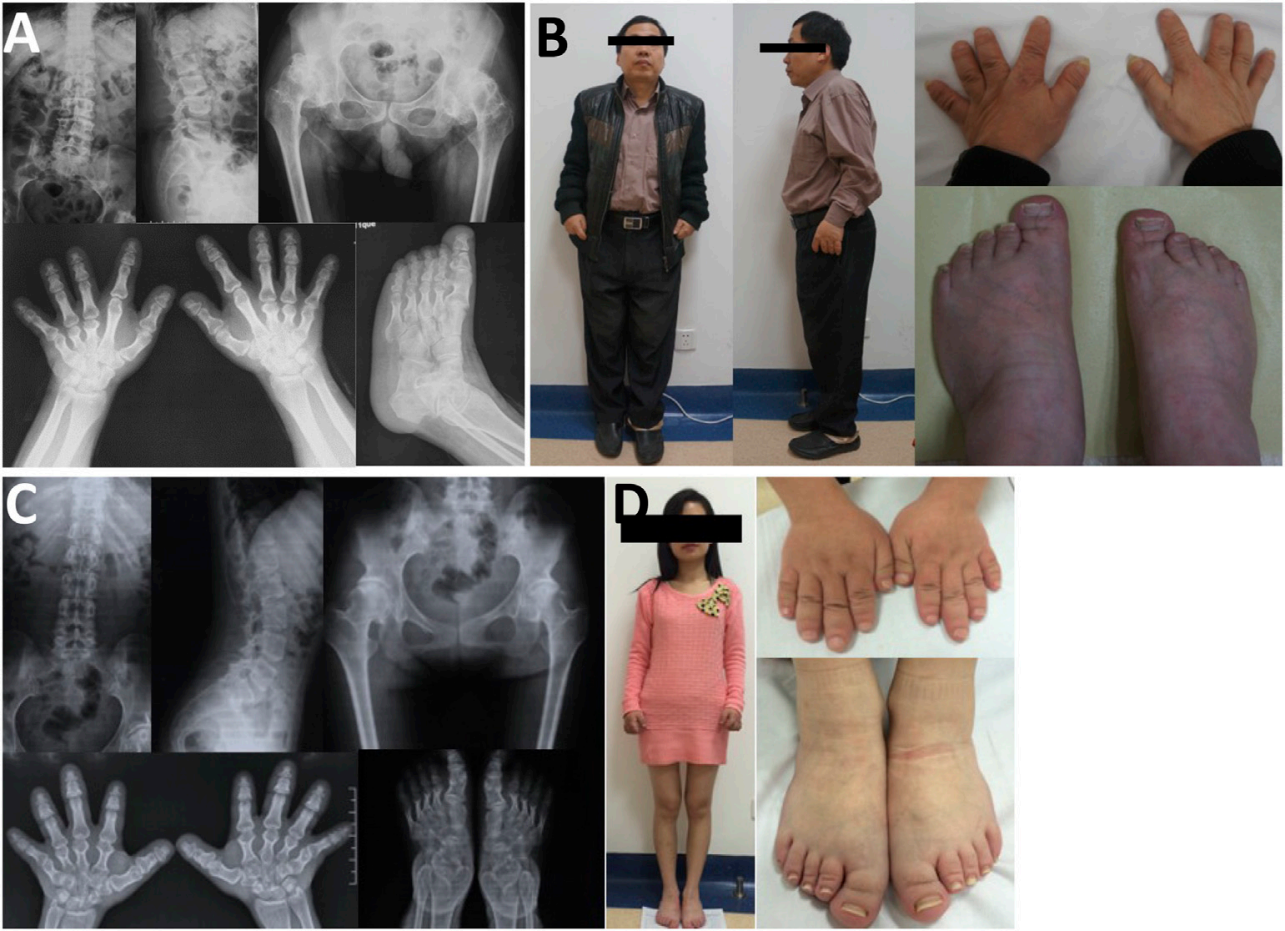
Photographs and radiographs of the family 7. (A,B) Radiographs of the spine, pelvis, hands, and feet (A) and photographs (B) of proband 7 (Ⅱ-2) in family 7. (A) Mild flattening and irregular shape of the vertebral bodies and 4–5 lumbar spondylolisthesis were shown in the spine. Flattening of the acetabulum, hip subluxation, femoral head necrosis, and shortening of the femoral neck were revealed in the pelvis. Widened epiphysis and joint cavity stenosis were displayed in the hands and feet. (B) Short stature with short limb was visible in the full body. Thick and short fingers and toes were shown in hands and feet. (C,D) Radiographs of the spine, pelvis, hands, and feet (C) and photographs (D) of patient (Ⅲ-1) in family 7. (C) Mild flattening and irregular shape of the vertebral bodies were displayed in the spine. The pelvis was normal. Widened epiphysis and joint cavity stenosis were displayed in the hands and feet. (D) Thick and short fingers and toes were shown in hands and feet.
